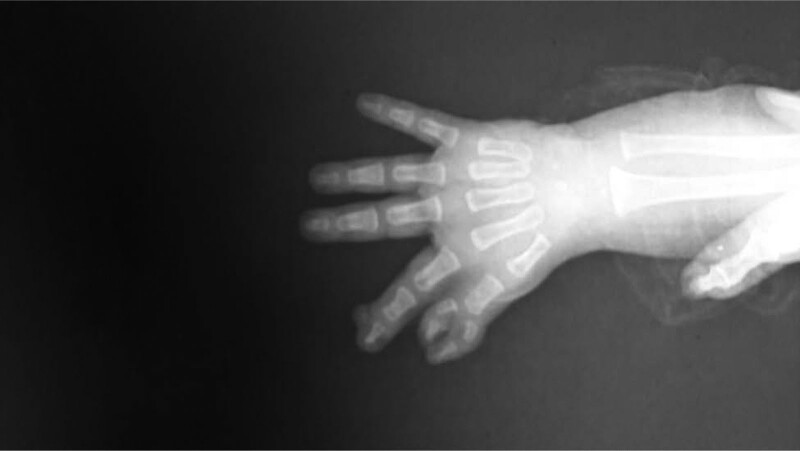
The picture X-rays of right hand that shows the deformities (triphalangeal thump, thumb duplication and syndactyly).
